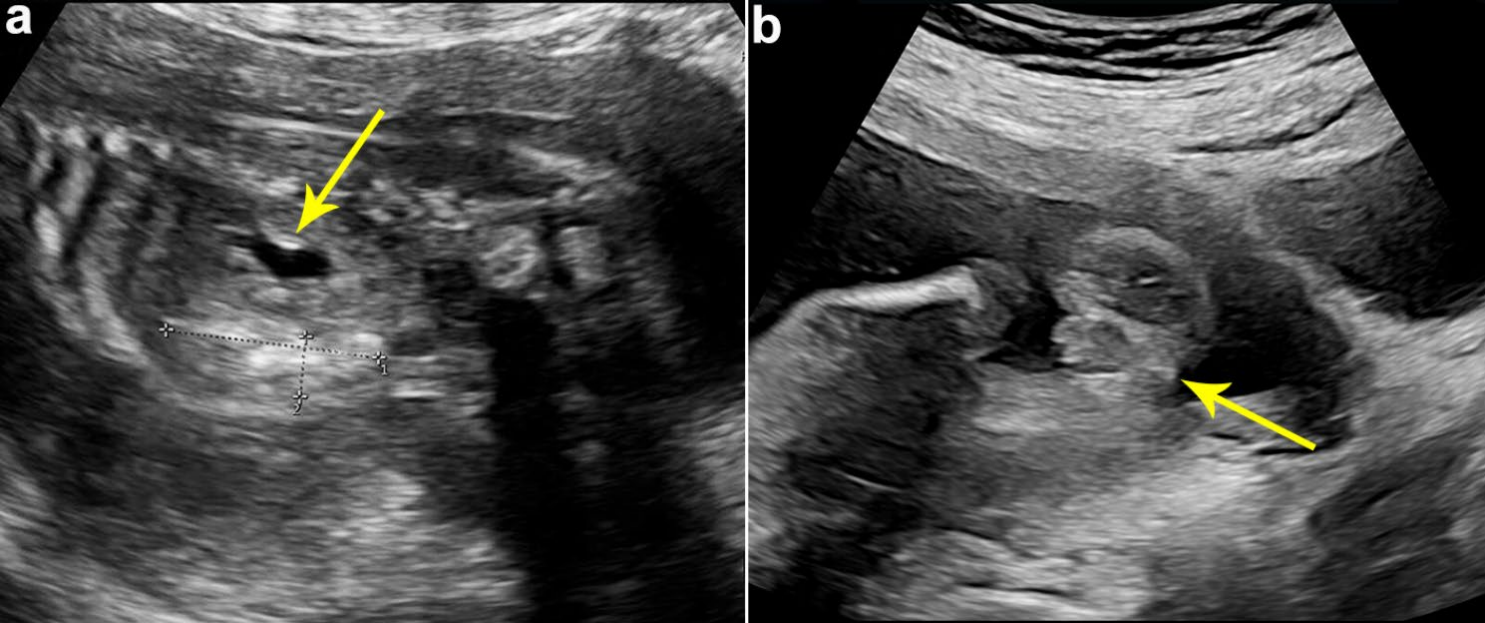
Ultrasound image at 23 weeks of gestation. (a) The echogenic bowel (cursors) and mild hydronephrosis of the left kidney (arrow). (b) The transverse sonogram of the perineum indicates the absence of a hypoechoic ring (arrow), suggesting the presence of an invisible anal sphincter
